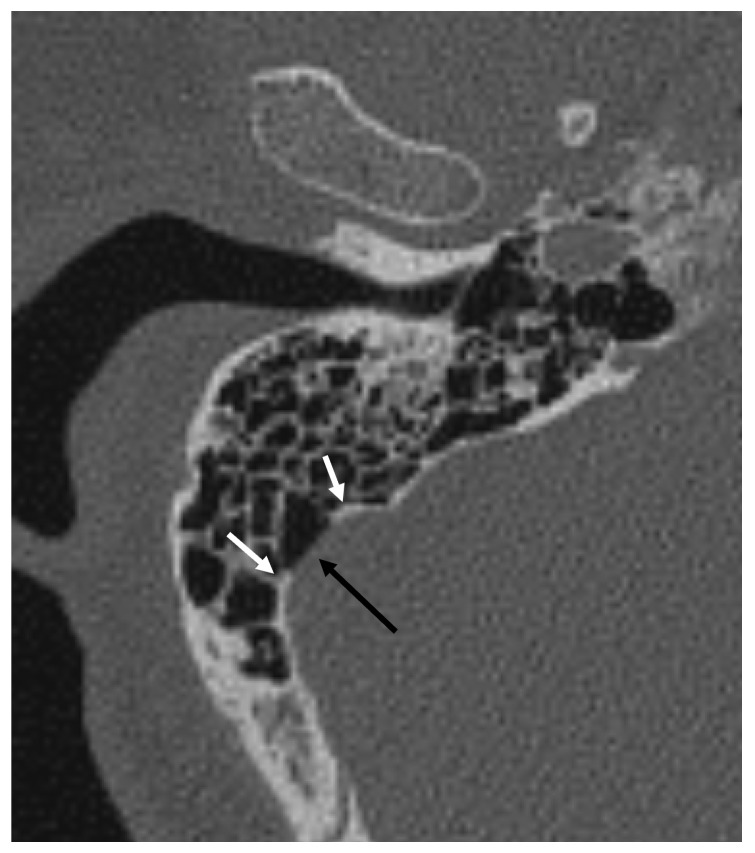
Axial CBCT image of the bony window shows a right dehiscent sigmoid sinus (black arrow). Inflection points of the sigmoid sulcus’s maximal curvature (white arrows) divide the wall into anterior, lateral and posterior ones.
